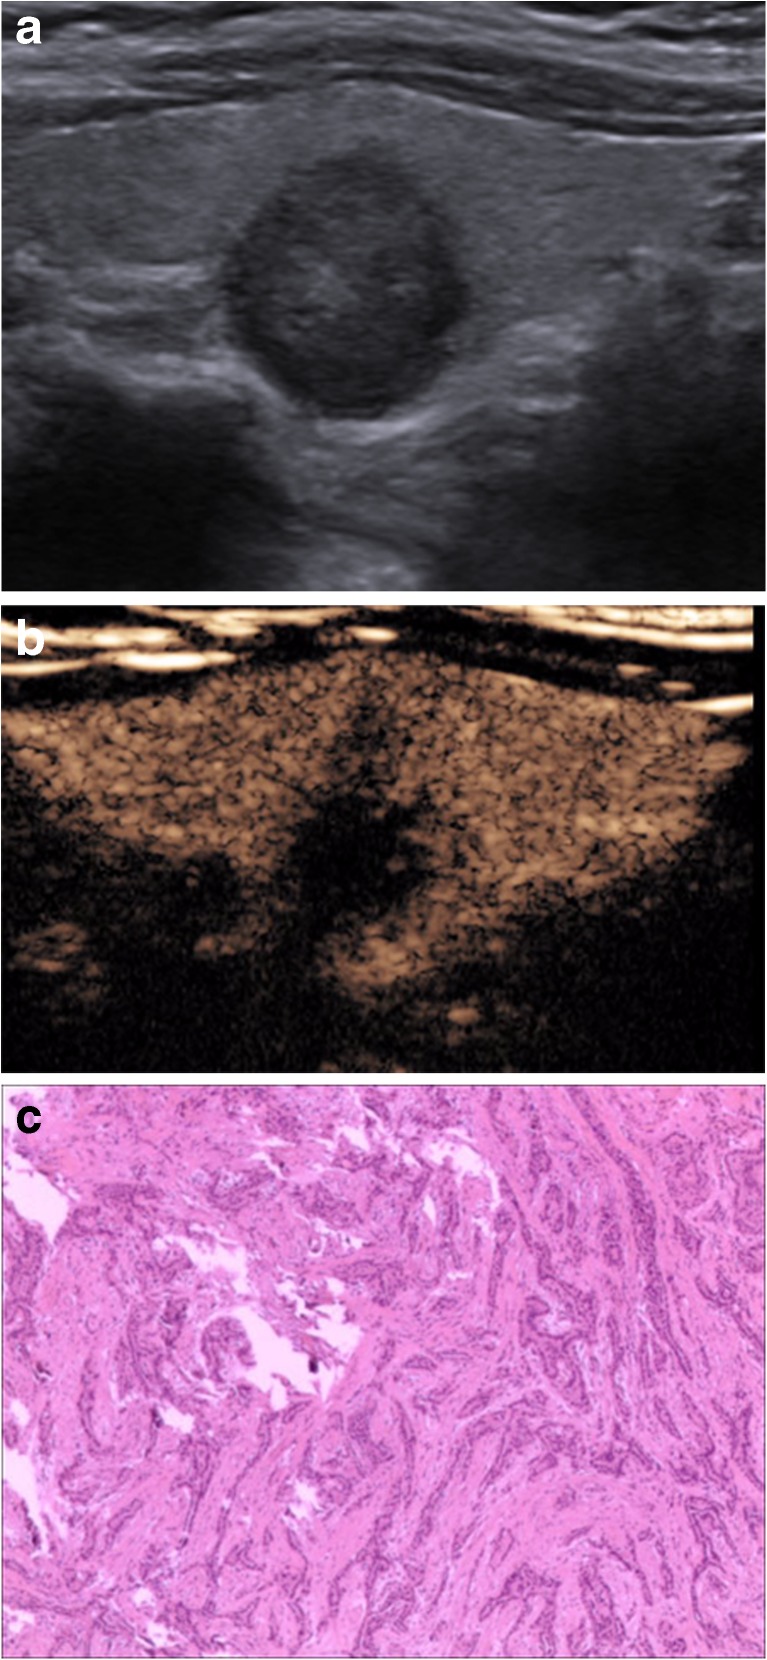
A 38-year-old woman was found to have a 12 × 12.5-mm solid hypoechoic nodule in the left lobe of her thyroid. a A conventional two-dimensional image. This nodule had three malignant indicators (solid, aspect ratio greater than 1 and markedly hypoechoic) and was classified to a 4b TI-RADS score. b An ultrasound contrast image; the enhancement mode was low enhancement. The improved TI-RADS combined with CEUS returned a score of 5, and the diagnosis indicates a malignant nodule. c The pathological image of the lesion, which is a thyroid papillary carcinoma
